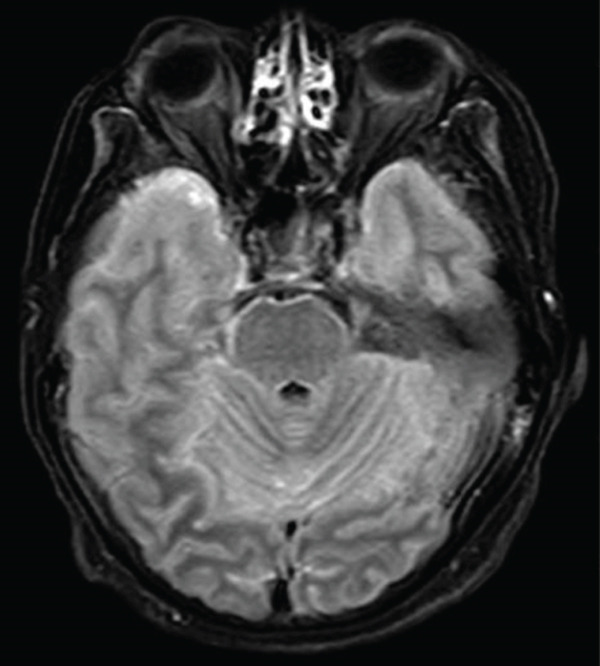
Linear enhancement in the right temporal pole on MRI.
